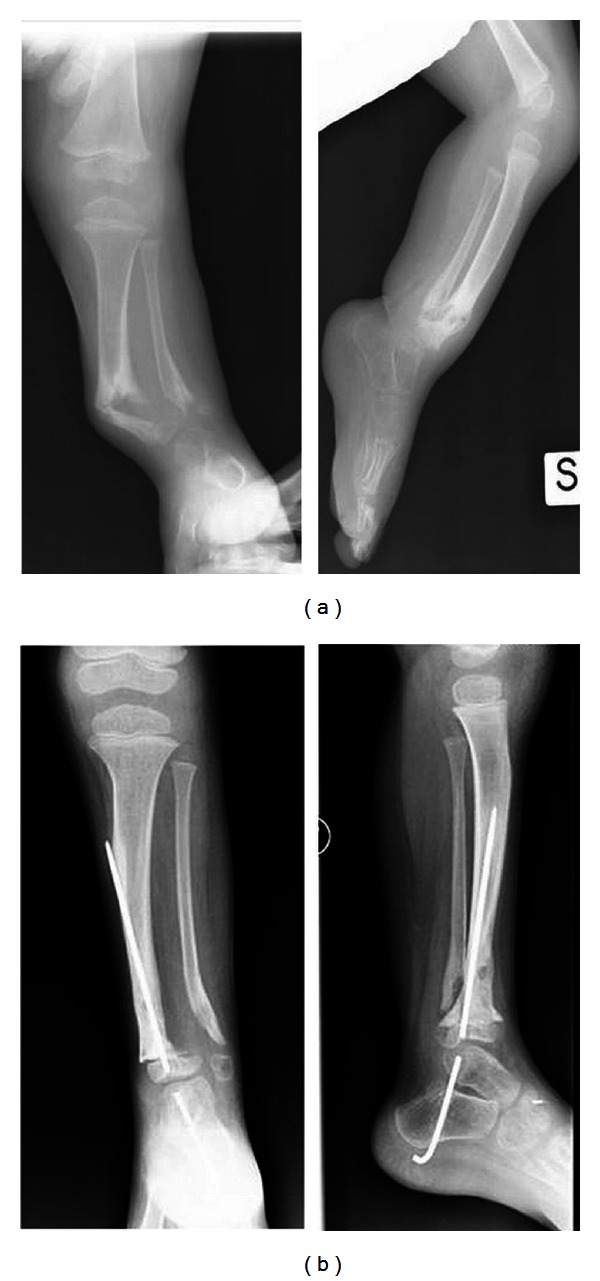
Clinical effect of the use of combined BM hMSC-based technique. Radiographics of a representative patient affected by NF1 with a tibial pseudarthrosis before (a) and three months (b) after the use of autologous BM hMSCs combined with platelet-rich fibrin and lyophilized bone.
